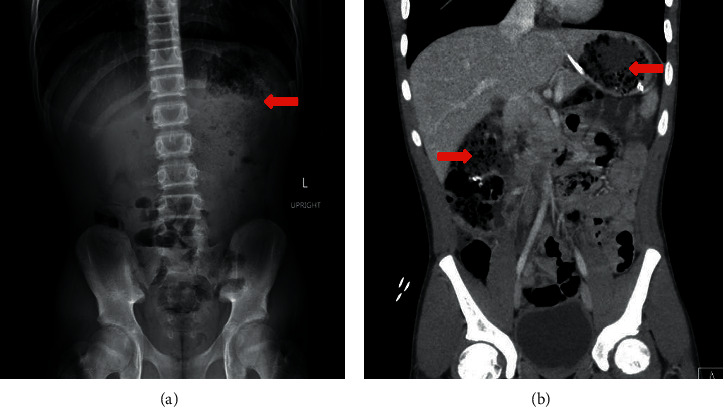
(a) Abdominal X-ray showing large amount of intra-gastric ingested material. (b) CT abdomen (coronal view) showing intra-gastric bezoar without gastric obstruction with similar contents in the proximal small bowel and ascending colon (red arrows).
